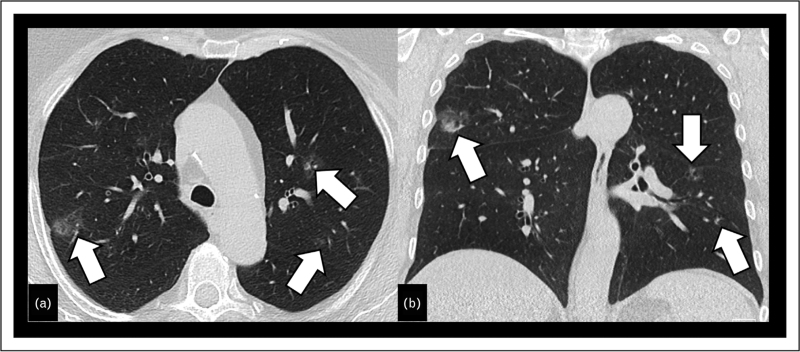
Transverse (a) and coronal (b) CT image reconstructions of the lung show multiple sub-solid nodules in both lungs. The largest lesion, located in the right upper lobe, measures 1.8 cm in total with a solid component measuring 0.6 cm (best appreciated on coronal reconstruction); there are three lesions (arrows), making it a T1a (3/m).
